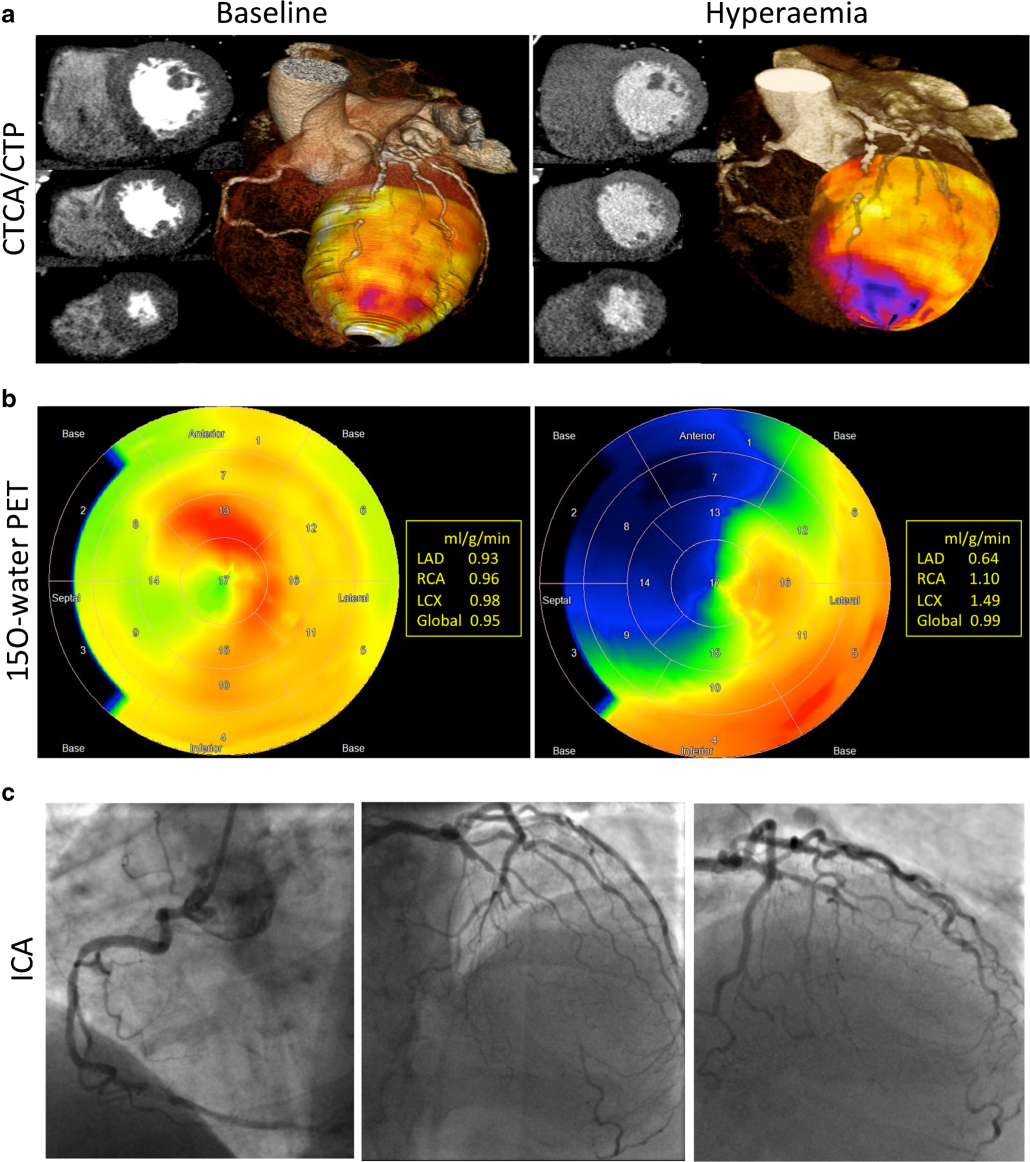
Example images from CT, PET and ICA. This 74-year-old non-smoker with hypertension, hypercholesterolaemia and atypical chest pain underwent multimodality assessment of his cardiac anatomy and physiology. a Short-axis basal, mid and apical images of the left ventricle at rest and during hyperaemia along with three-dimensional representations of the transmyocardial perfusion ratio and coronary anatomy. Hypoattenutation is seen in right coronary artery (RCA) and left anterior descending (LAD) artery during hyperaemia. There is also mild hypoattenuation in the RCA territory on rest imaging. b Corresponding ^15^O-water PET images which identified the same perfusion abnormalities in terms of the absolute myocardial blood flow (mL/(g/min)). c Corresponding images from ICA which identified an occluded LAD and severe stenosis of the RCA with FFR less than 0.80
